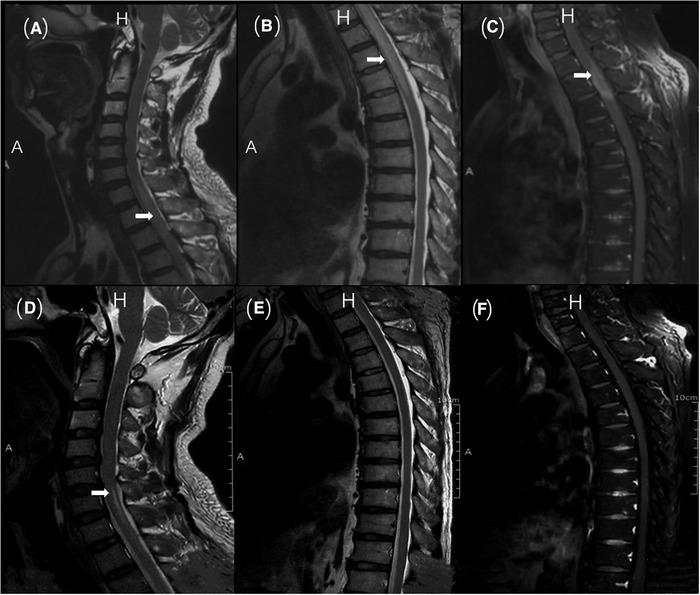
Spine cord MRI of the patient. The spine cord MRI (A–C) shows continuous T2 hyperintensity lesion extending from C6 to T5, with focal patchy gadolinium enhancement at T1–T3. Sagittal T2 image of the cervical spine (A). Sagittal T2 image of the thoracic spine (B). Sagittal T1 image of the thoracic spine postinfusion of gadolinium, the arrowhead indicates the area of gadolinium enhancement (C). A total recovery of the spinal cord swelling and a few T2 hyperintensity with no enhancement 1 month later (D–F). Sagittal T2 image of the cervical spine (D). Sagittal T2 image of the thoracic spine (E). Sagittal T1 image of the thoracic spine postinfusion of gadolinium (F). A indicates anterior and H indicates head
